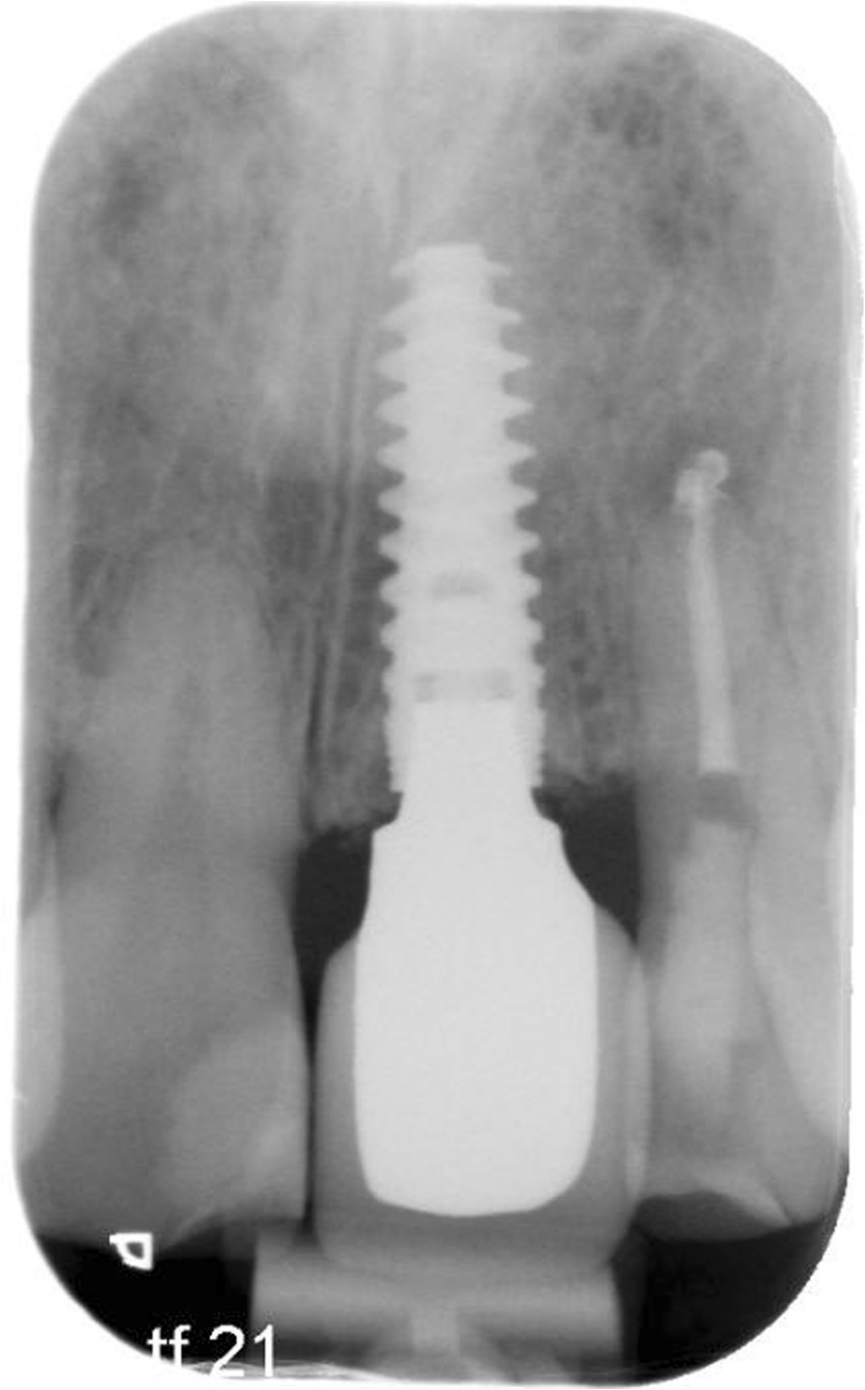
Conventional intra-oral radiograph of same patient with implant-supported restoration at position 21.
